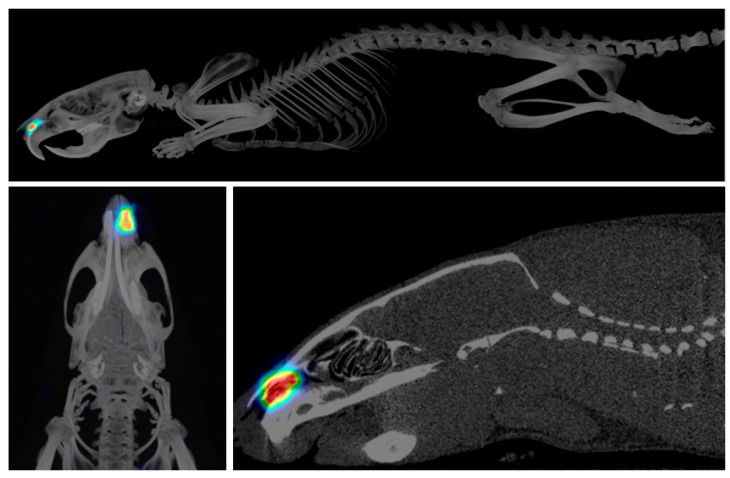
Fused MicroSPECT-CT images showing the location of the microimplant inside the nasal cavity. Hotter colours indicates higher concentration of radioactivity.
